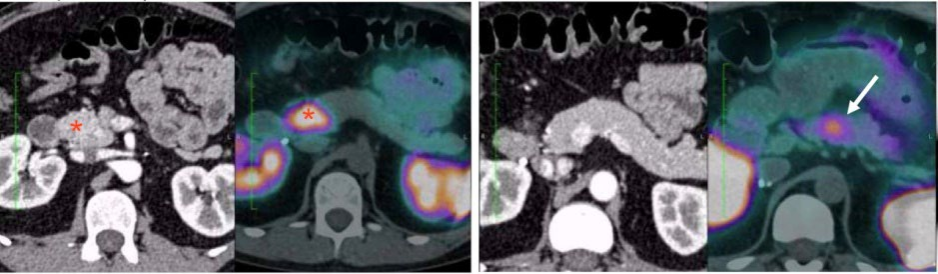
Positron emission tomography-computed tomography scan with DOTATOC-68Ga. Left: the uncinate process tumor in the computed tomography scan with the corresponding image on the Positron emission tomography-computed tomography (*). Right: the pancreatic body tumor is not viewable on the computed tomography scan but detected with the Positron emission tomography-computed tomography (arrow).
